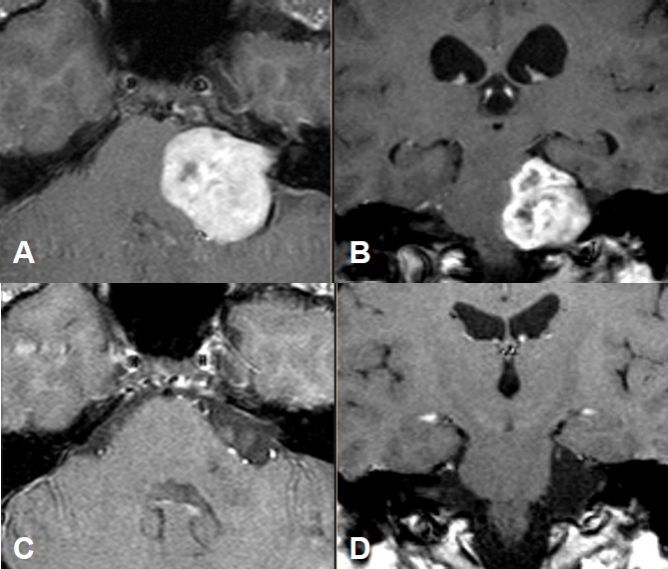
Preoperative MRI, T1-w + CM; A transversal; B coronal. 1 year after surgery, MRI, T1-w + CM; C transversal; D coronal; gliosis zone in the left cerebellar peduncle as consequence of the compression by the tumor. The hydrocephalus regressed, the ventricular system is again normally wide.
